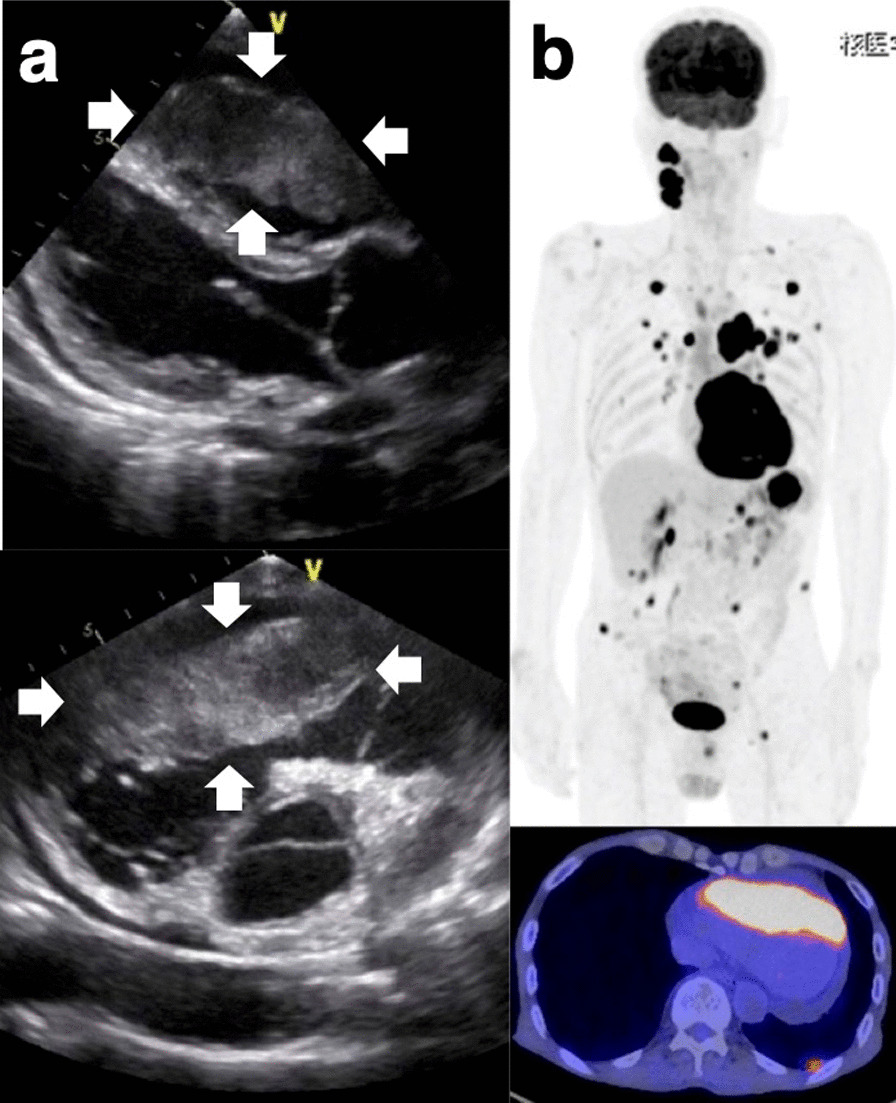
Imaging findings. a An echocardiogram showed the presence of a large immobile mass attached to the patient’s right ventricle extending to the outflow tract. b An FDG-PET showed multiple foci of abnormal FDG accumulation in right cervical and mediastinal lymph nodes, cardiac wall, and stomach
